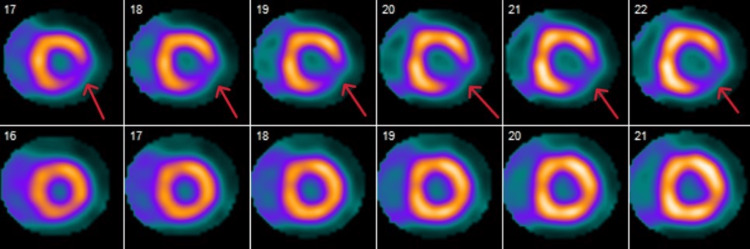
Basal short-axis view of PET CT with arrows pointing to areas of ischemia in the basal inferolateral region consistent with mid-left circumflex and obtuse marginal 1 artery occlusion.
